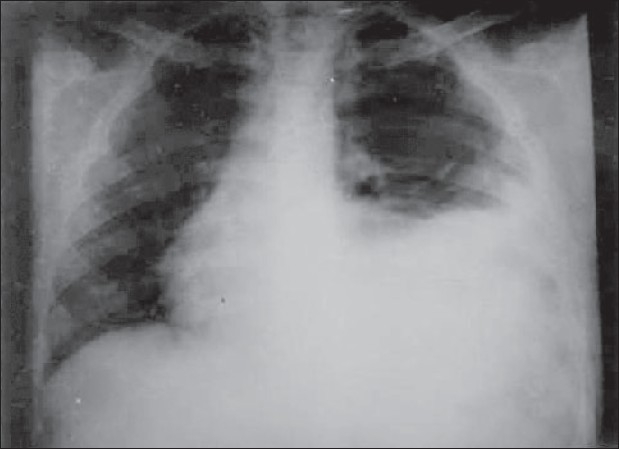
Chest radiograph on which child was treated for pulmonary tuberculosis
